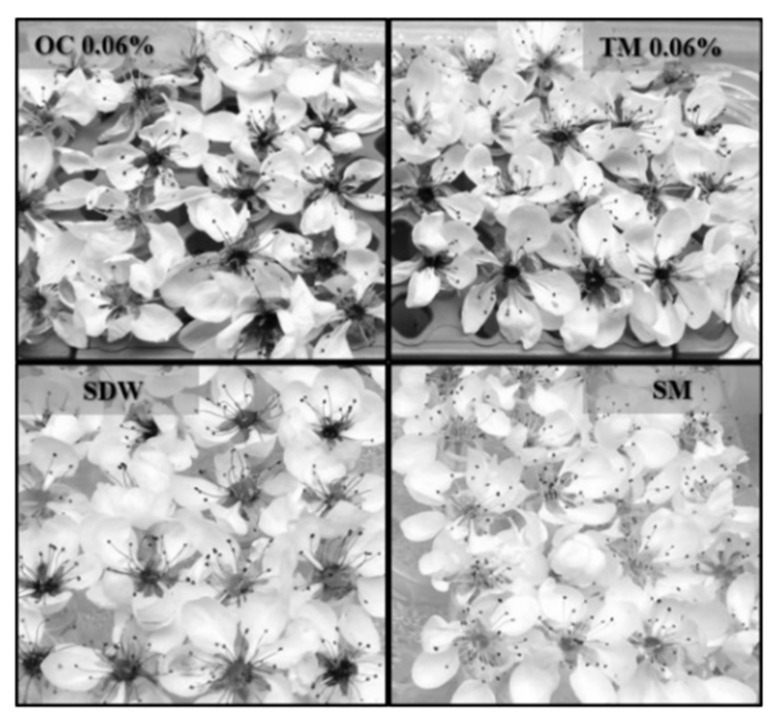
Fire blight incidence on pear flowers cv. Williams. Treatment: *O. compactum* CT carvacrol at 0.06% (OC 0.06%), *T. vulgaris* CT Thymol at 0.06% (TM 0.06%), streptomycin sulphate at 100 μg/mL (SM, positive control), sterile distilled water (SDW, negative control).
